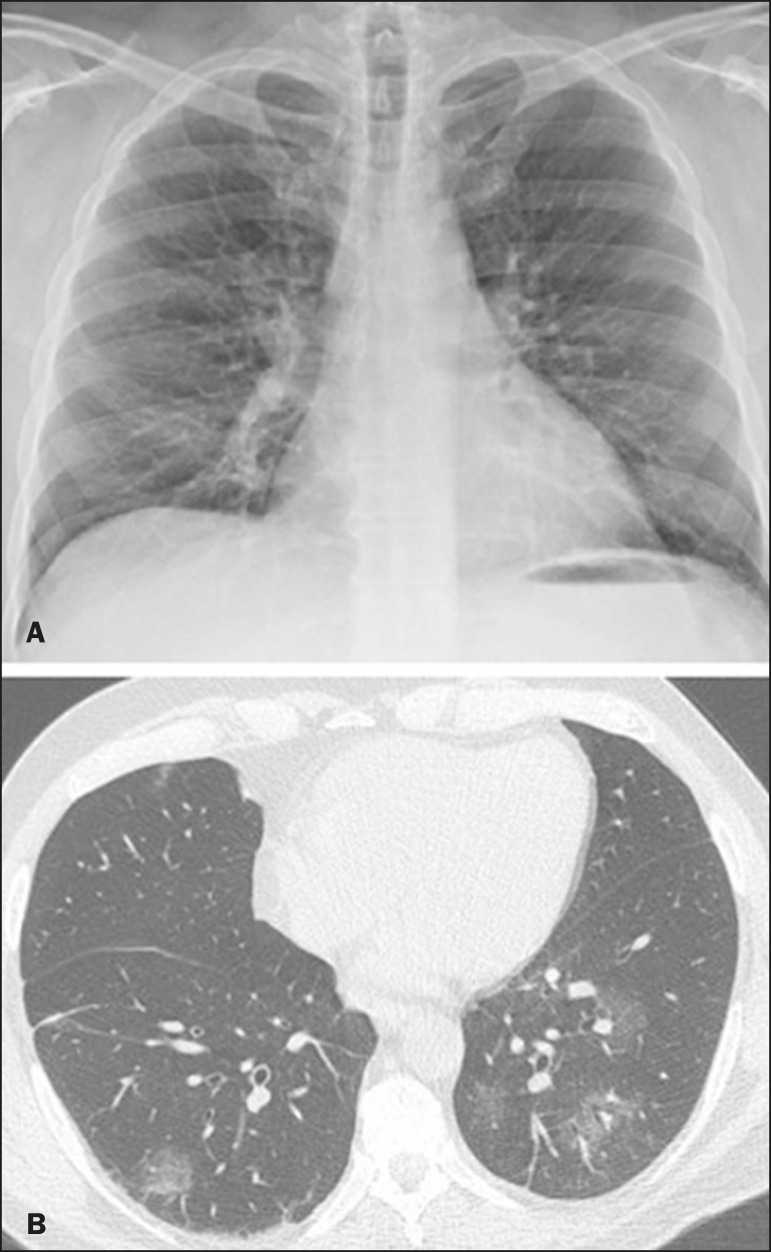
Male patient, 35 years old. Posteroanterior chest radiography (A) showing no pulmonary changes. MDCT (B) on the same day shows opacities with ground-glass attenuation in the lower lobes. Positive RT-PCR for SARS-CoV-2.
